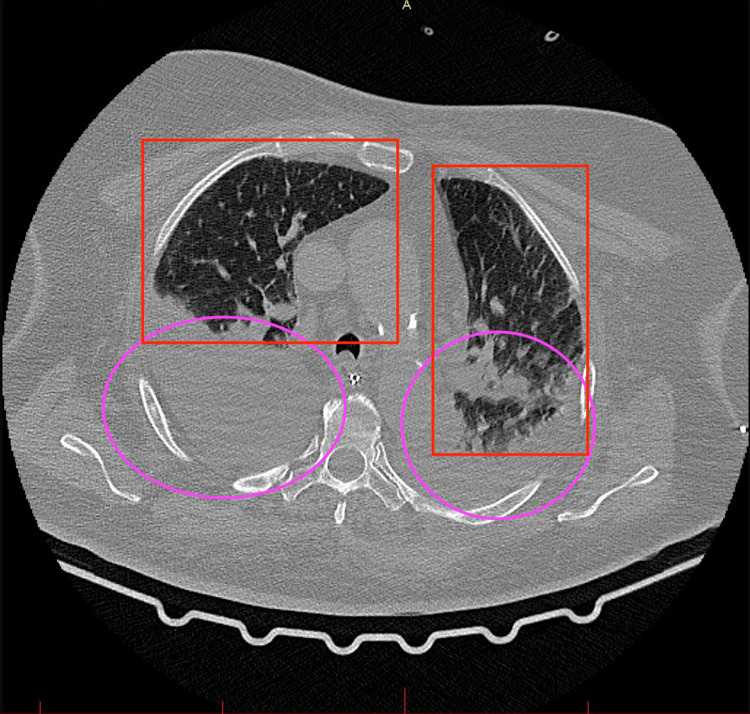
CT scan of the lungs. Lower pulmonary lobes Pink circles: presence of fluid; Red rectangles: typical inflammatory image of lungs after COVID-19 infection
